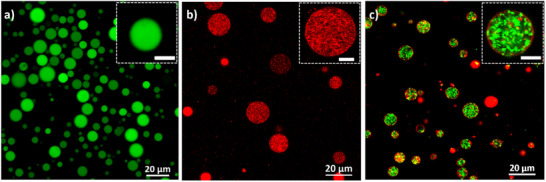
Cargo‐loaded GUV analysis. GUVs containing a) calcein (green signal), b) 100 nm SUVs (red signal: LissRhod PE in SUV membrane); and c) 200 nm fluorescent beads (green signal: beads; red signal: LissRhod PE in GUV membrane). The insets in the top right corner of each image show a magnified view of a single GUV from each sample. Scale bars in the insets are 5 µm.
